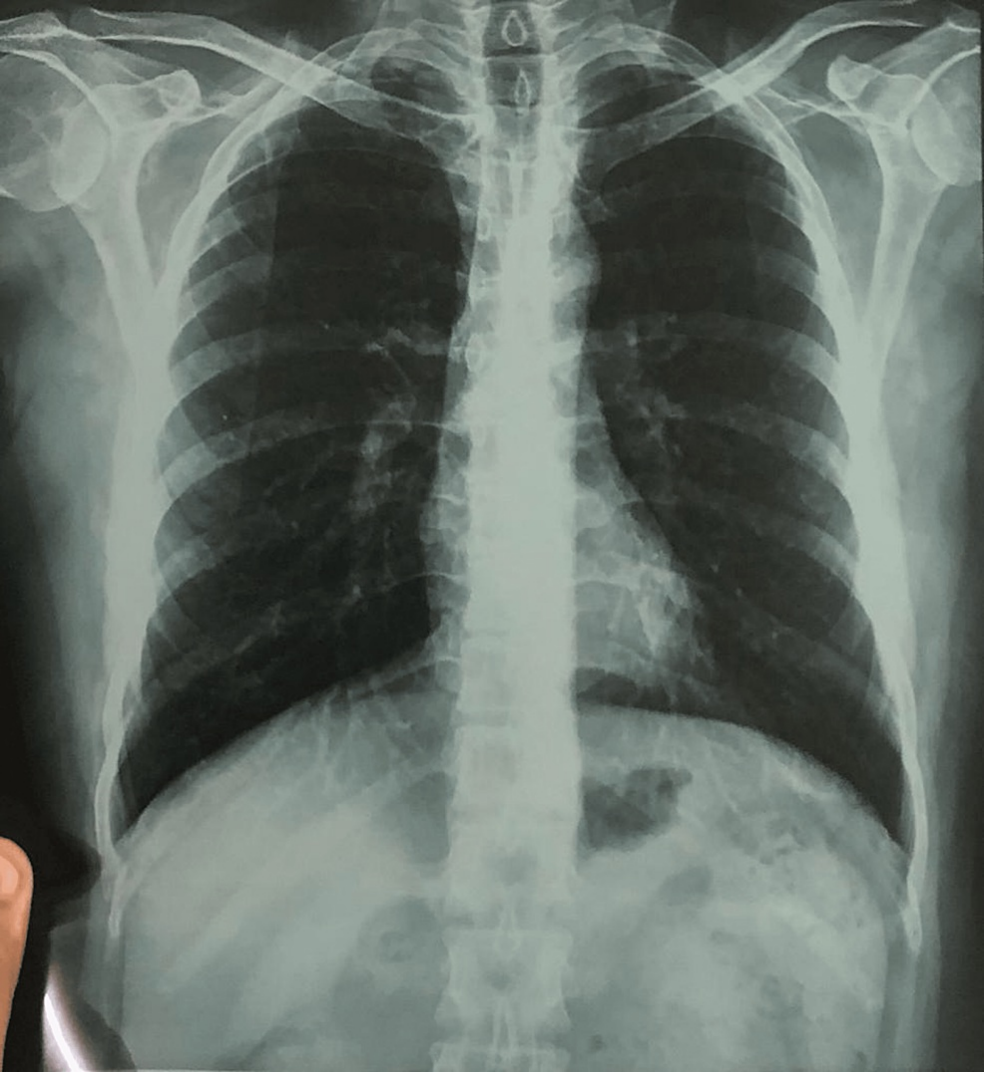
X-ray chest posteroanterior view showing a centrally placed trachea, normal cardiothoracic shadow, no mediastinal widening, and no osteopenia, masses, or infiltrates
